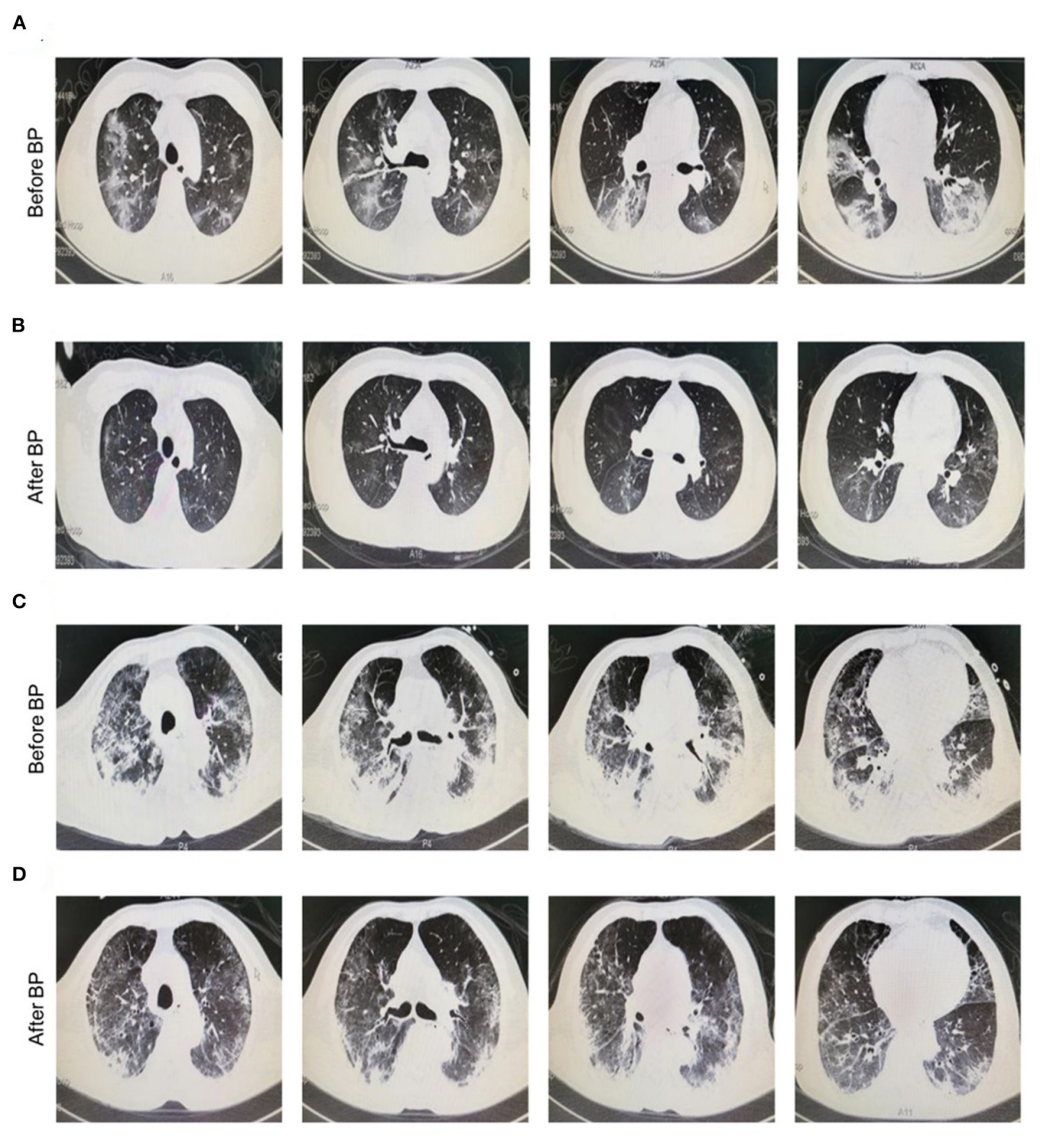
Chest radiographs of COVID-19 patients before and after blood purification. (A,B) Representative chest CT manifestation of a 35 year old male patient with severe COVID-19 infection before (A) and after (B) blood purification treatment. This patient was diagnosed with dyspnea (FiO2, 41%, SpO2, 90%) and received HFNC and blood purification via CVVH/CVVHDF for one time. Finally, he was discharged from hospital with negative result of nuclei acid test (NAT). The typical ground-glass lesion as well as the inflammatory exudate in the lung of the case with severe COVID-19 infection before and after blood purification indicated the favorable prognosis. (C,D) Representative chest CT manifestation of a 75 year old male patient with critical COVID-19 infection before (C) and after (D) blood purification treatment. The lung of the patient with infiltrating ground-glass opacity in imaging before blood purification was significantly alleviated after treatment. The patient was diagnosed with ARDS, MODS, COPD and had comorbidities of type II diabetes and CHD. Before and after blood purification, the patient was positive and negative for NAT for SARS-CoV-2 in pharyngeal swabs, nasal swabs, stools and saliva, and turned negative after treatment, respectively.
